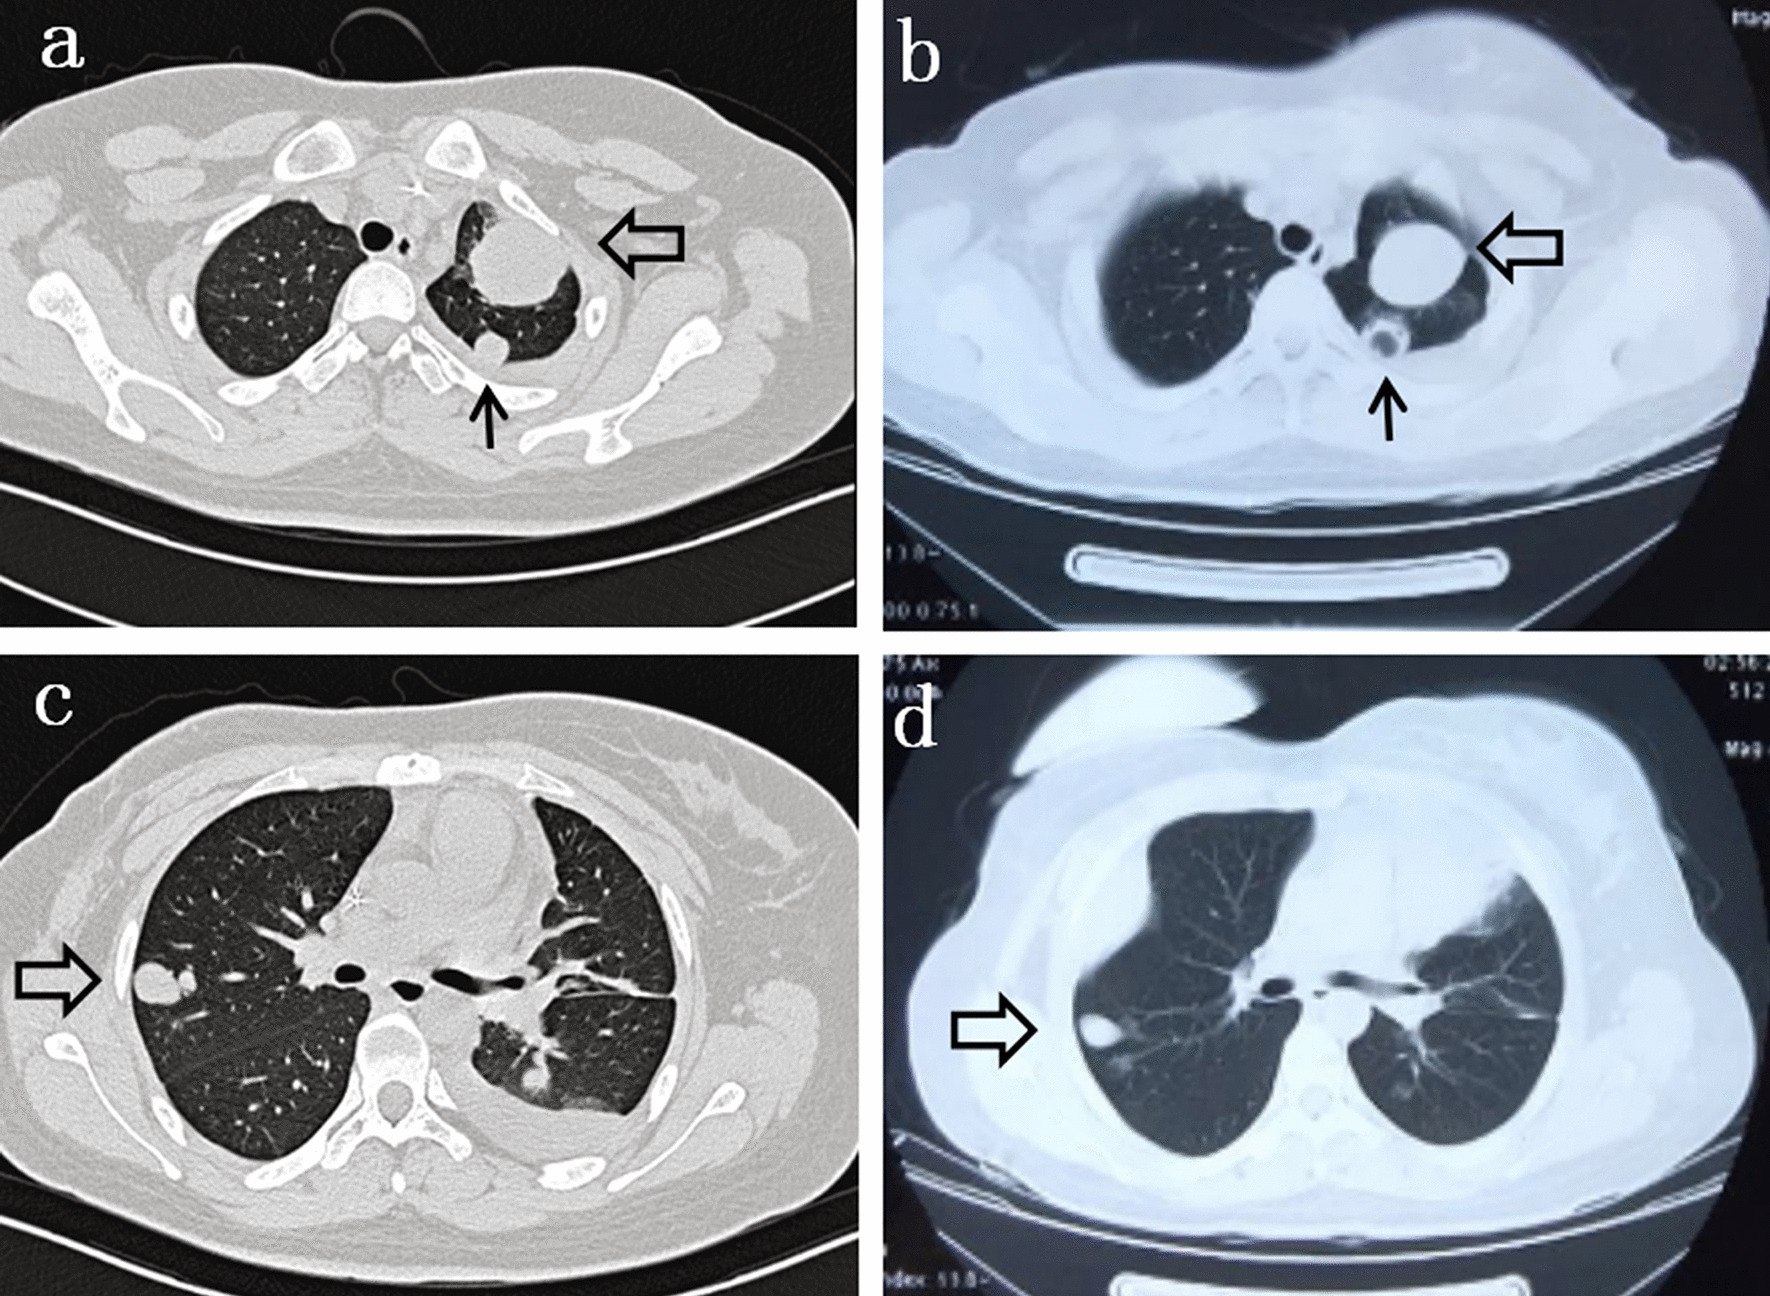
Chest CT scans before and after apatinib therapy. a Before apatinib therapy (March 09, 2018), a CT scan showed solid nodules. b After 2 months of apatinib treatment (May 11, 2018), one of the solid nodules was reduced (hollow arrows) and the other one transformed to a cystic nodule (black arrows). c Before apatinib therapy (March 09, 2018), a CT scan showed the mass (hollow arrow). d After 2 months of apatinib treatment (May 11, 2018), the mass was smaller than 2 months before (reduced in size by 15%)
